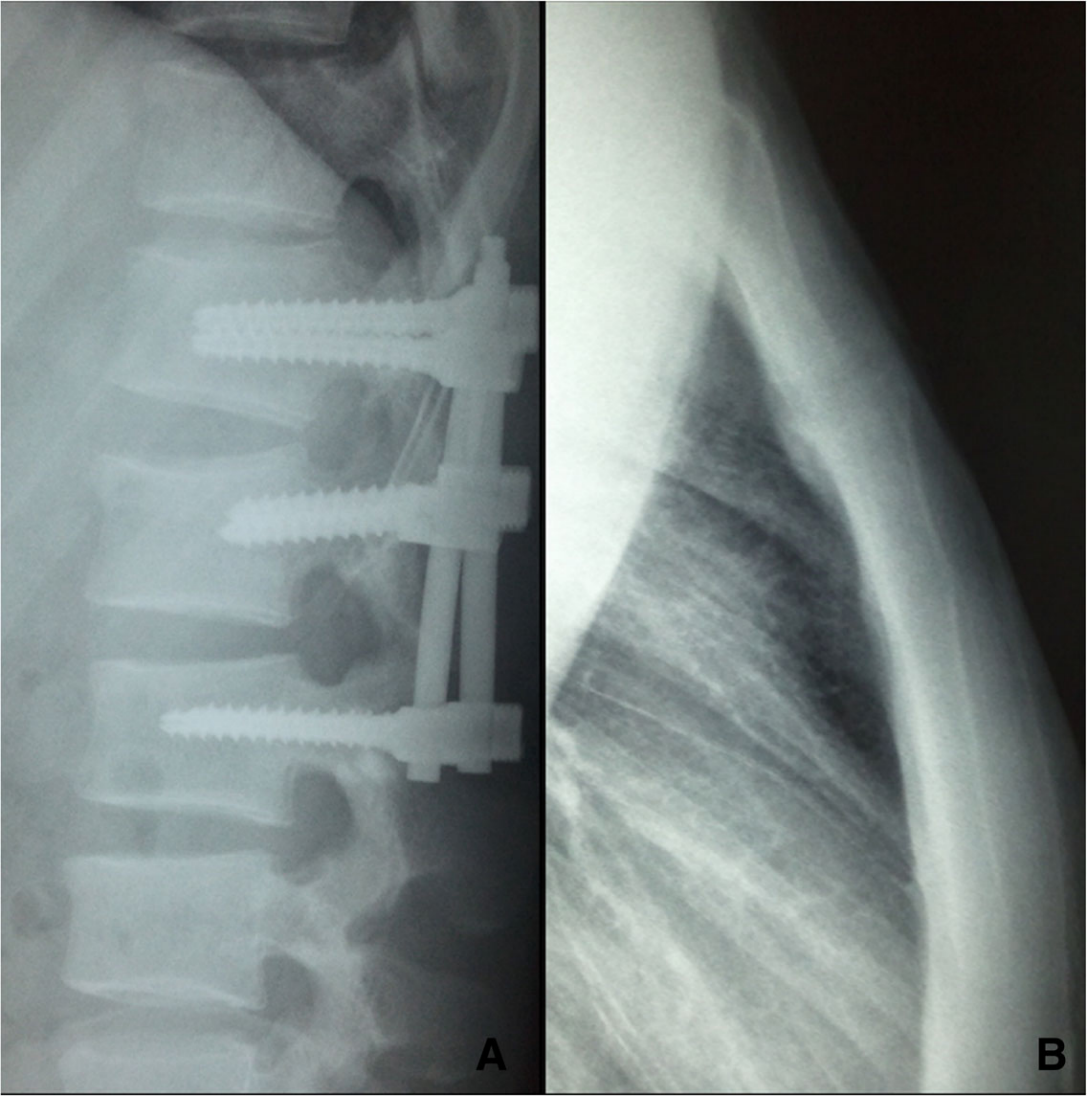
a Lateral radiograph showed fracture healing of the lumber and b malunion of manubriosternal dislocation, including appearance deformity of sternum
